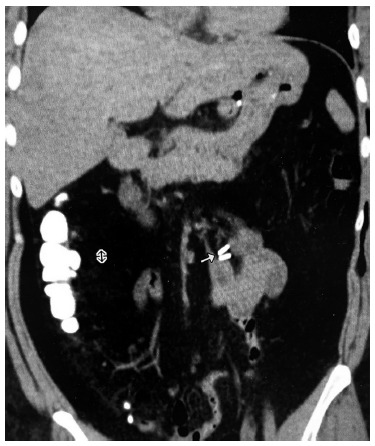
Abdominal CT scan: clips in normal position, to the left of the spine in case of obstruction in the anastomosis, not Petersen’s hernia, which regressed with conservative treatment (control CT revealing contrast in the ascending colon).
